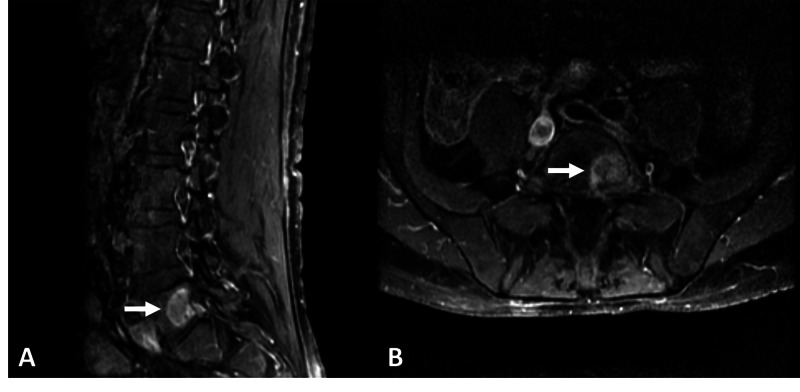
Lumbar spinal metastasis. Sagittal (A) and axial (B) T1 post-contrast enhancement MRI of a large ring enhancing lesion invading the left, posterolateral aspect of the L5 vertebral body
